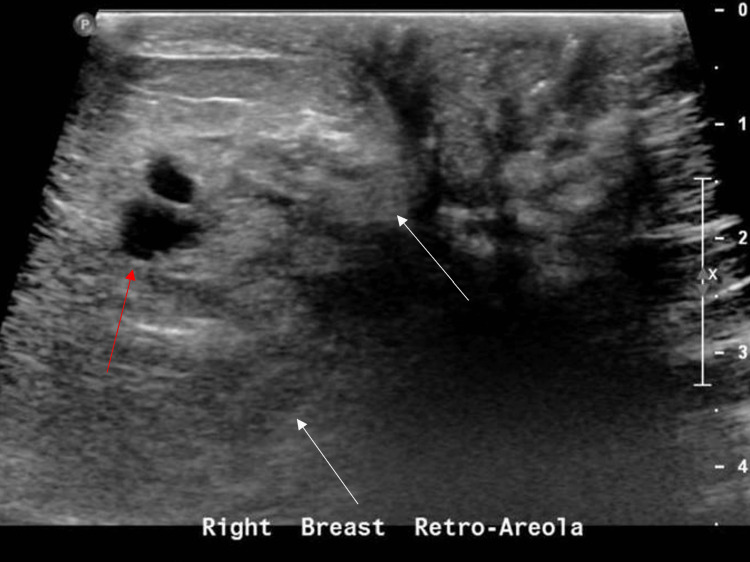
Ultrasound of the right breast retro-areola showing moderate swelling as well as heterogenous breast changes (white arrows). Focal areas of questionable nodules are seen in the inner aspect (red arrows).
